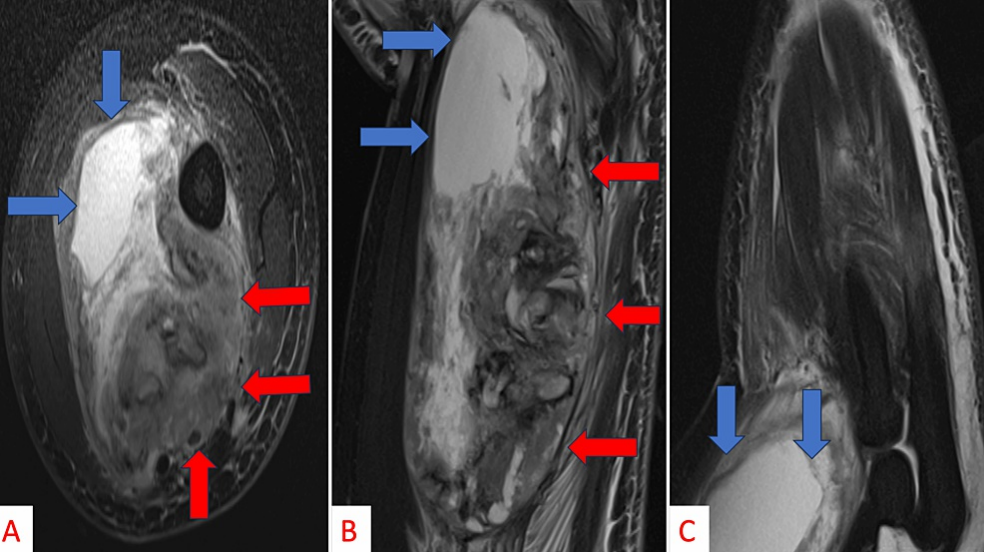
Axial (A), coronal (B), and sagittal (C) MRI imaging displaying a heterogenous mass with irregular enhancing nodular soft tissue and a large cystic/necrotic component consistent with a soft tissue sarcoma (red arrows indicate borders of the pseudocapsule, blue arrows indicate peritumoral edematous fluid accumulation).
